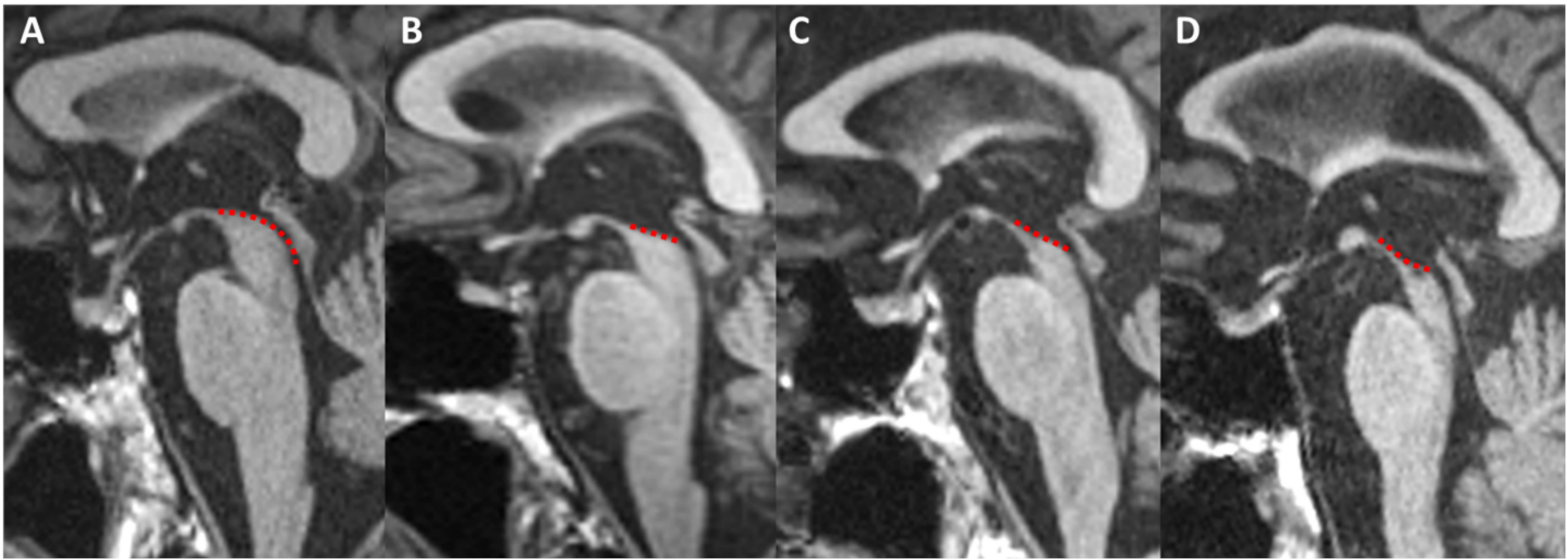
Midbrain atrophy in PSP patients. Midsagittal T1-weighted images in a healthy control (A) and PSP patients with increasing midbrain atrophy (B–D). Healthy subjects and PD patients show a normal convex profile of the midbrain superior surface. PSP patients present a flat (mild) or concave (moderate to severe) aspect (dashed line). This shape is known as the “penguin” or “hummingbird” sign. HC, healthy control; PD, Parkinson's disease; PSP, progressive supranuclear palsy.
